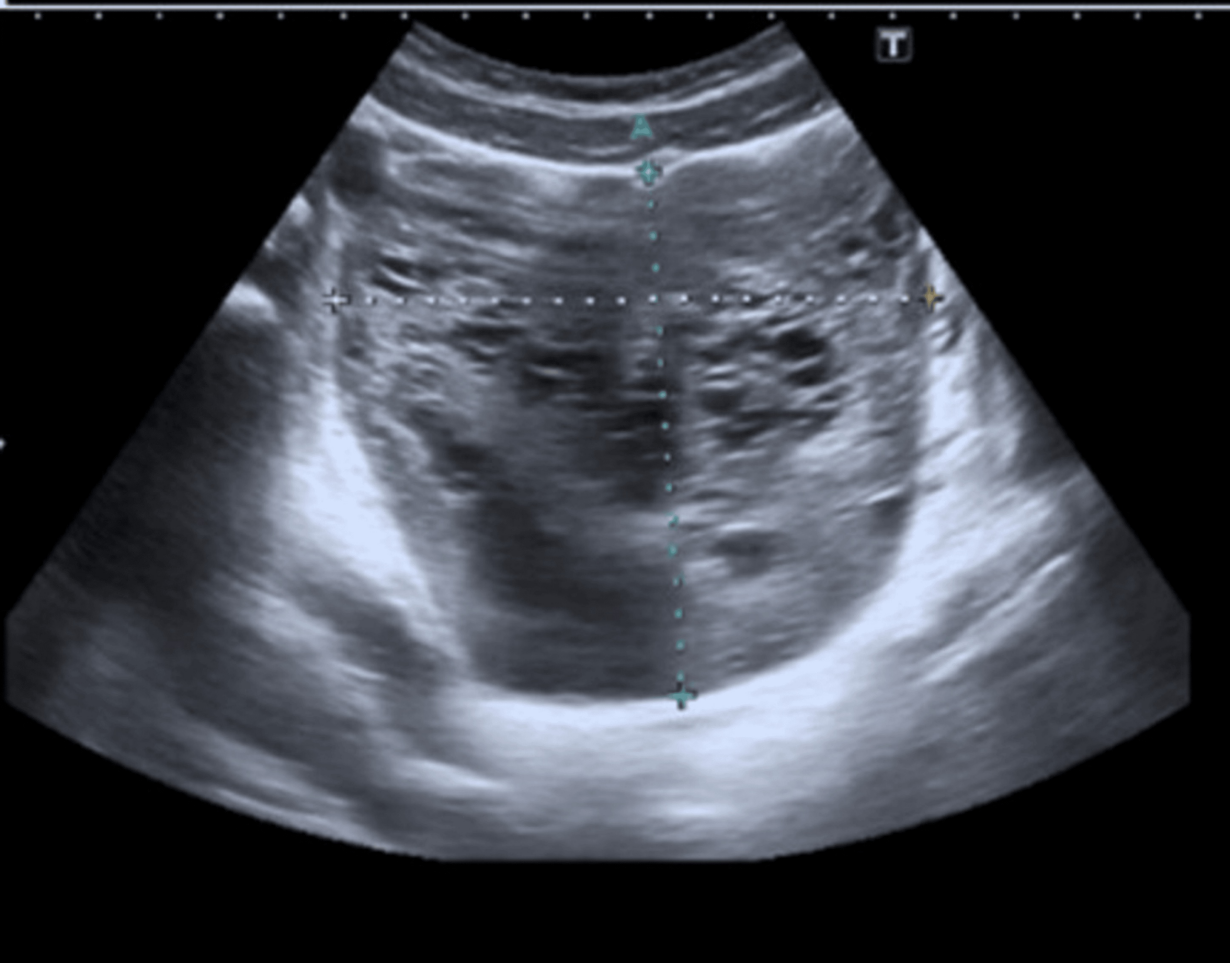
Transabdominal ultrasound scan showing adnexal mass
